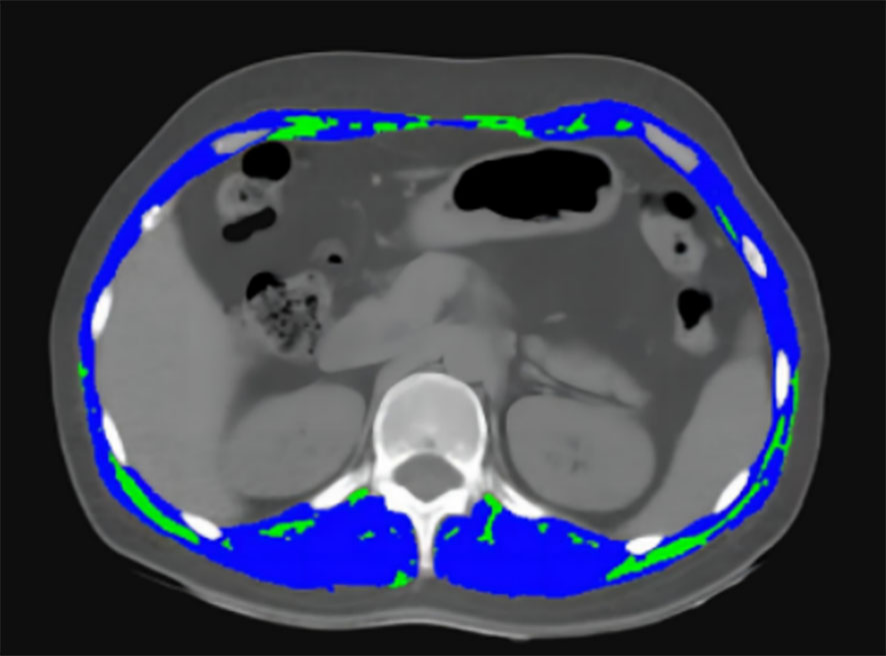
The image depicts the transverse section at the level of T12 on chest CT images. Sarcopenia was measured using the combined area of multiple skeletal muscles at T12, including the rectus abdominis, external oblique, internal oblique, latissimus dorsi, intercostal, and erector spinae muscles (blue). The intramuscular adipose tissue (IMAT) was showed as green area.
